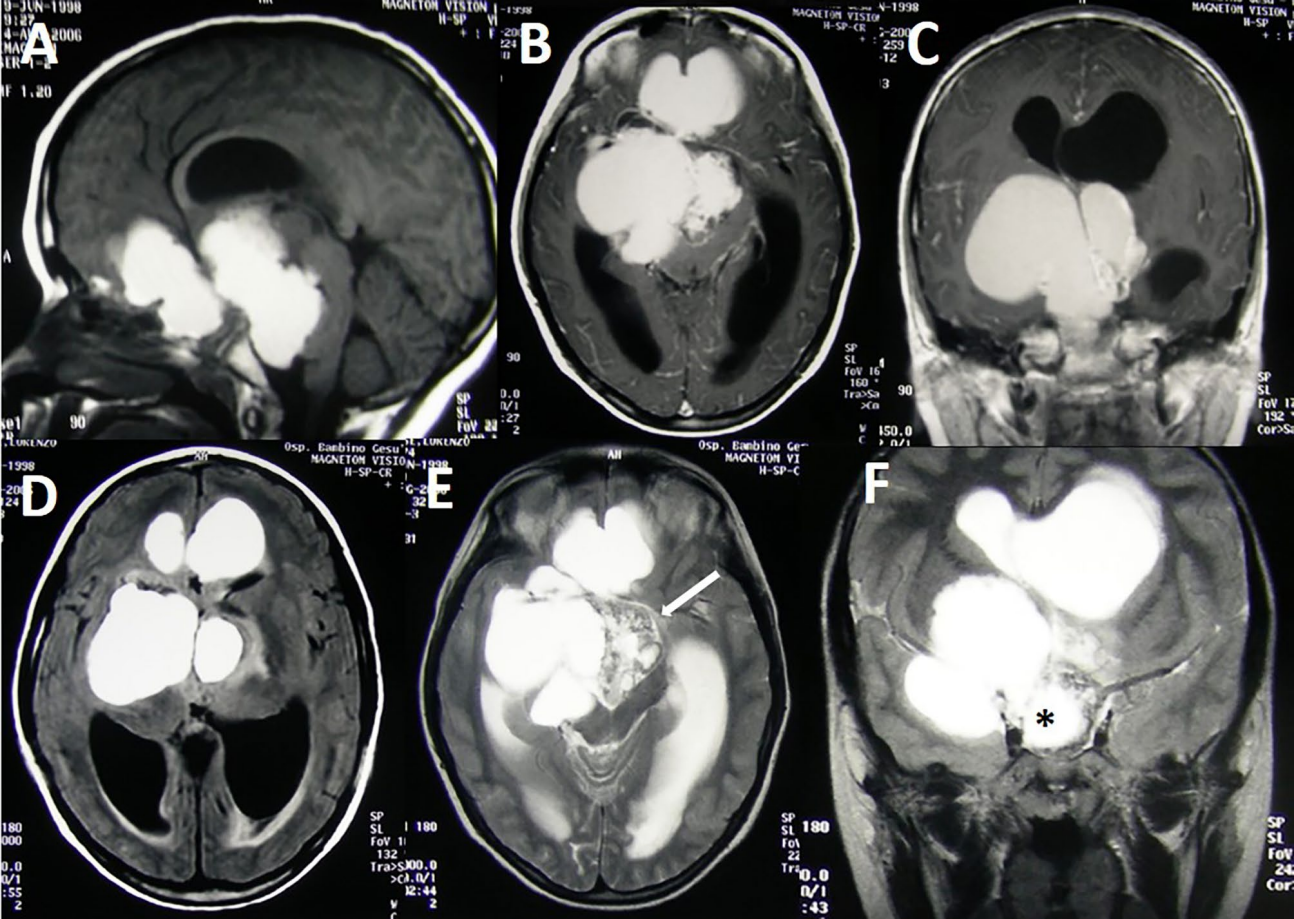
Giant AC in a 11-year-old boy. Note the large and enhancing cystic components in the T1 sequences after gadolinium administration (A, B, C), which extend into the anterior, middle, and posterior cranial fossa. Also a biventricular hydrocephalus is evident (A–E). The cyst fluid is hyperintense compared to CSF in FLAIR sequence (D). Note that the solid component is a minor part of the tumor (E, arrow) but involves the region of the Willis’ circle (F, asterisk)
